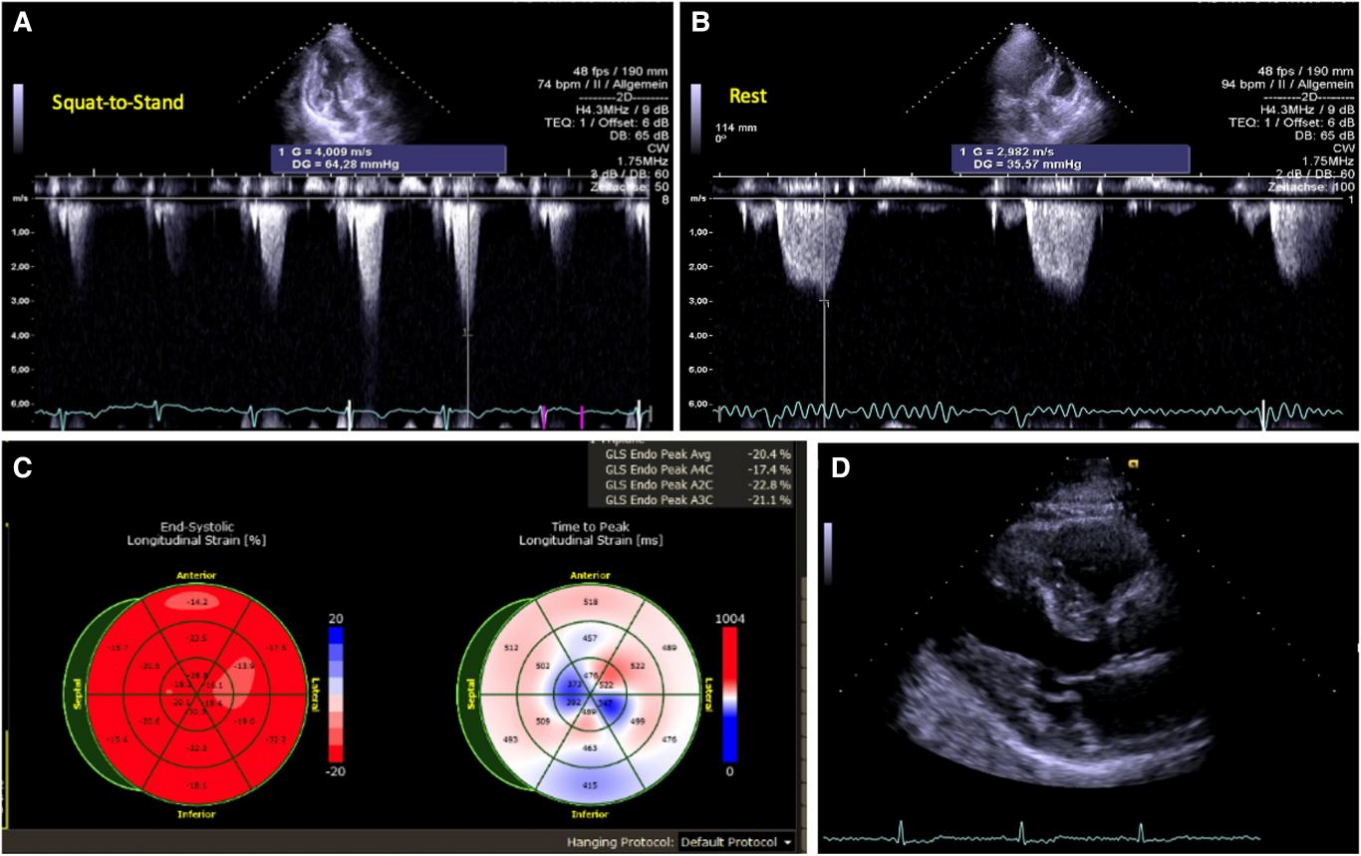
Echocardiography showed hypertrophy of the left ventricle with a maximum thickness of the left ventricular septum of 20 mm and of the posterior wall of 12 mm (*D*). Left ventricular ejection fraction was 77%, and myocardial deformation analysis did not indicate significant relative apical sparing or reduced global longitudinal strain in the predominant hypertrophic area (*C*). A resting gradient of 36 mmHg (*B*) with no change during Valsalva manoeuvre, and a gradient of 64 mmHg at squat-to-stand manoeuvre (*A*) was measured.
